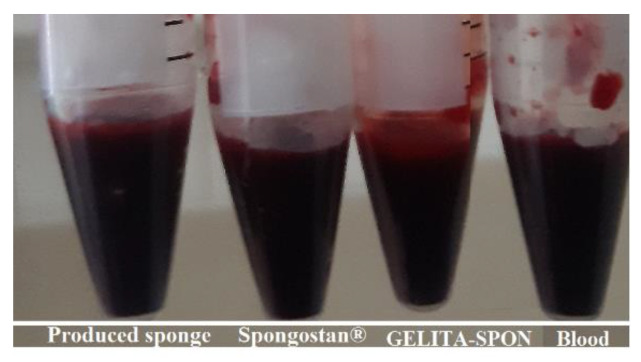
The results for CT coagulation time.
